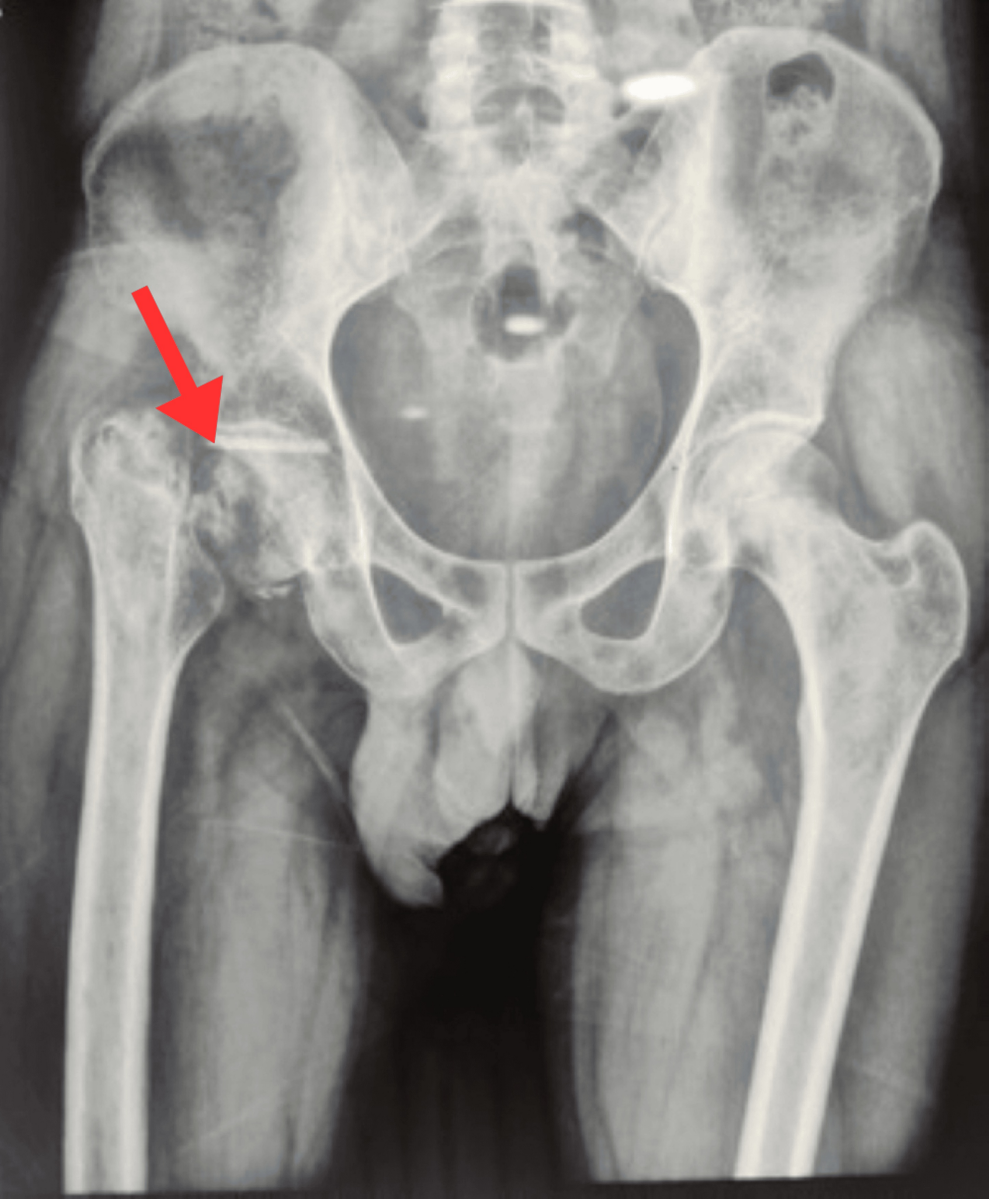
Preoperative radiograph The red arrow indicates non-union of the femoral neck with proximal migration of the greater trochanter.
